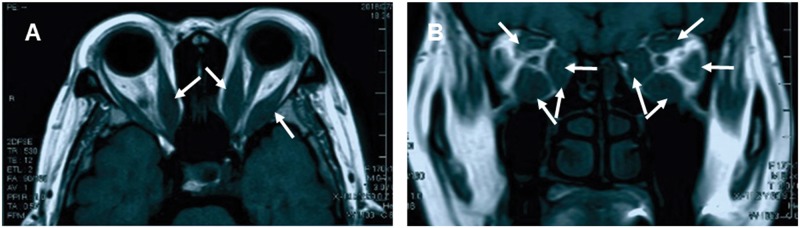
Severe involvement of extraocular muscles on magnetic resonance imaging scan of a patient with TAO. (A) Axial scan demonstrates the fusiform enlargement of extraocular muscles of a patient with thyroid associated ophthalmopathy (white arrows). (B) Coronal scan shows the involvement of muscle belly on magnetic resonance imaging scan of a patient with thyroid associated ophthalmopathy (white arrows).
